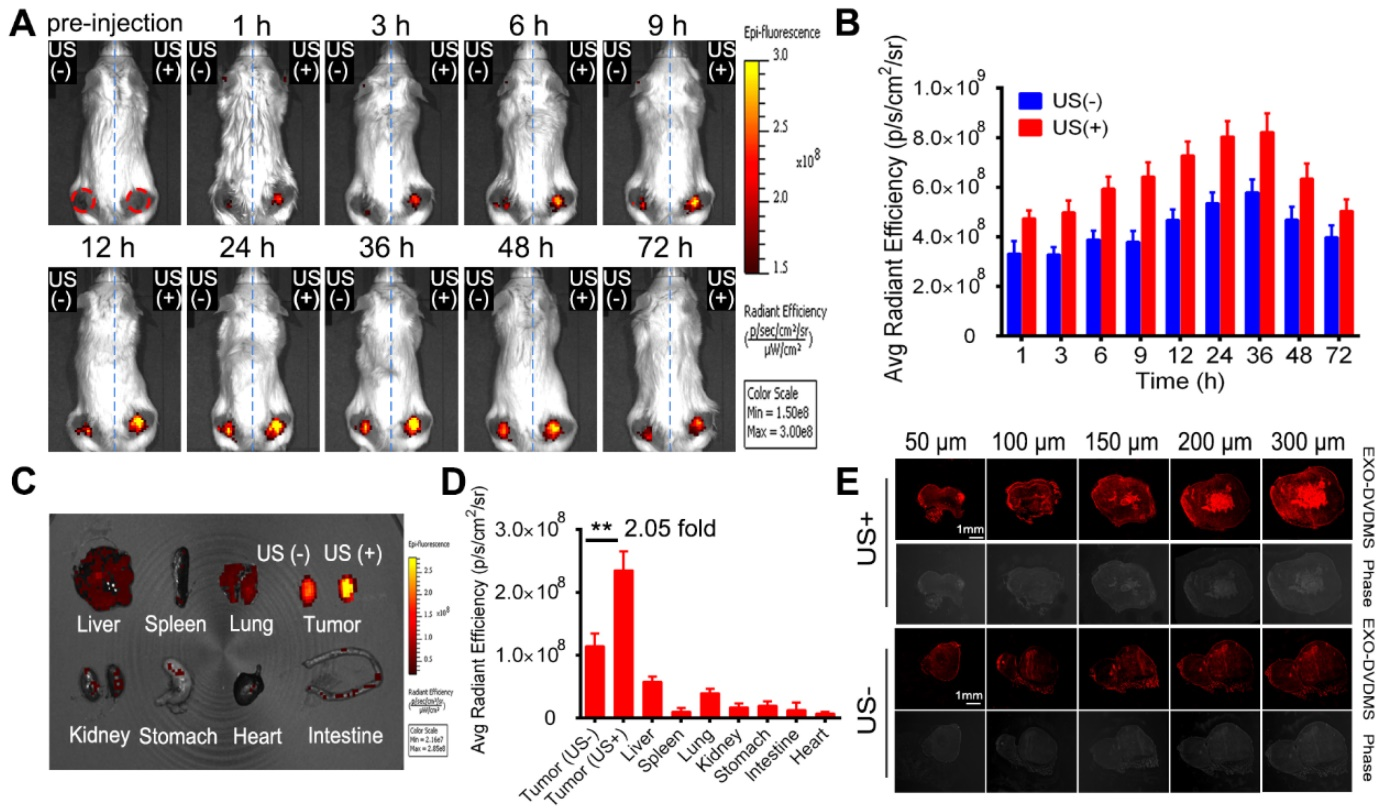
In vivo* bio-distribution and ultrasound promoted *in situ* EXO-DVDMS deposition and permeation. (A)
